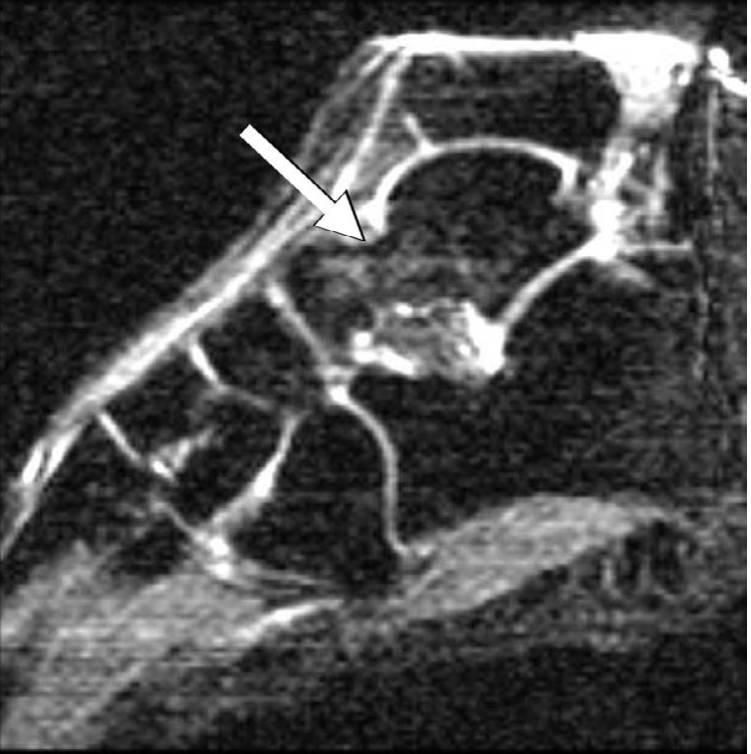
Sagittal STIR MR image of a 32-year-old female shows patchy edema signal within the talar neck (arrow).
